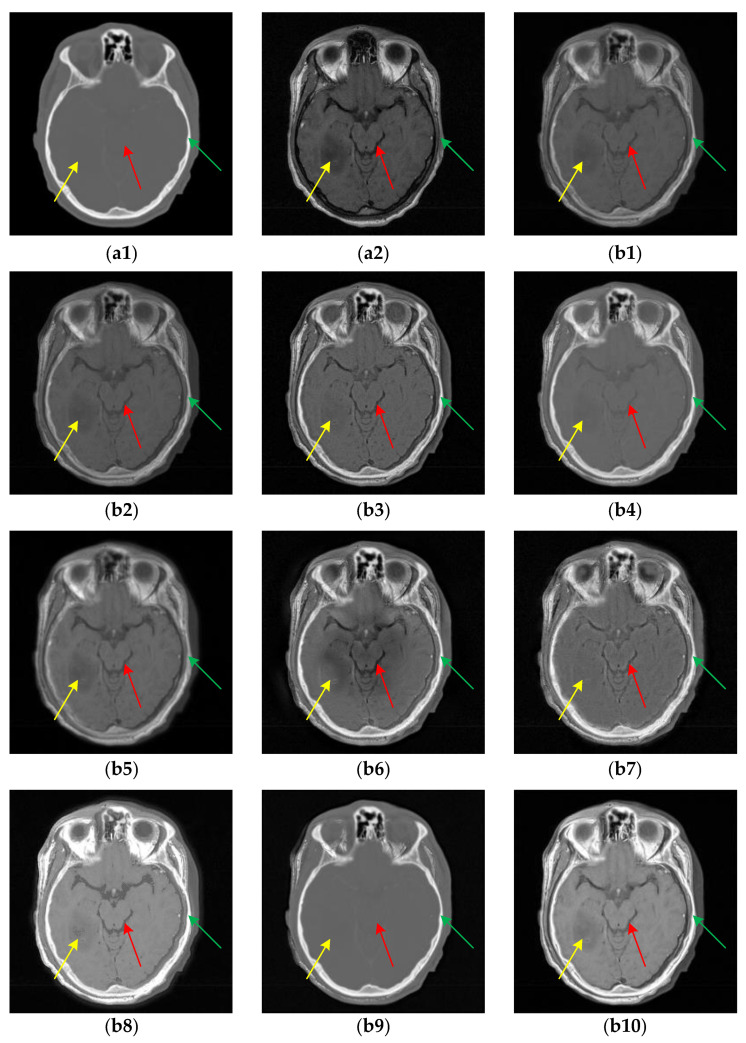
The results of all algorithms on the CT-MR image pairs from RIRE dataset. (a1) Source CT image; (a2) Source MR image; (b1) U2Fusion; (b2) DenseFuse; (b3) IFCNN; (b4) NestFuse; (b5) RFN-Nest; (b6) PAPCNN; (b7) ReLP; (b8) TIF; (b9) CDDFuse; (b10) CIRF.
